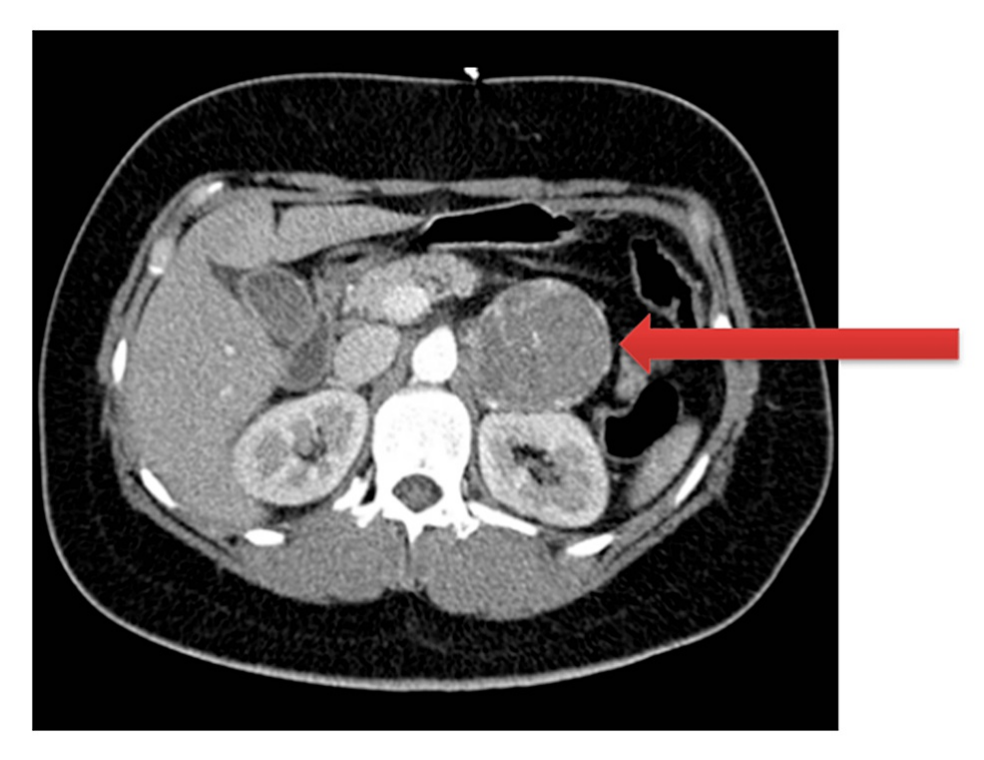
Computed tomography scan of the abdomen, showing the paraganglioma (red arrow)
